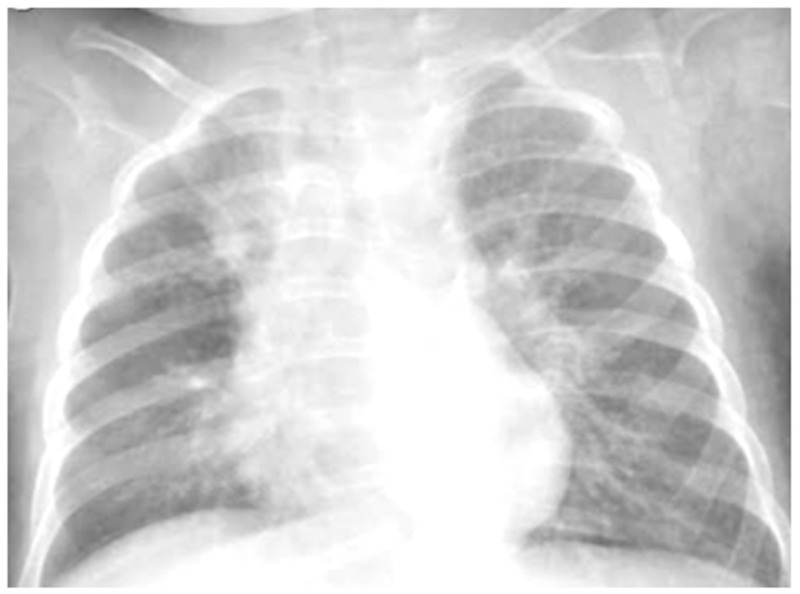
Chest radiography shows, on the right fields in the apical and basal areas, a non-specific area of reduced transparency. It also shows signs of interstitial engagement in the para-hilar position bilaterally.
